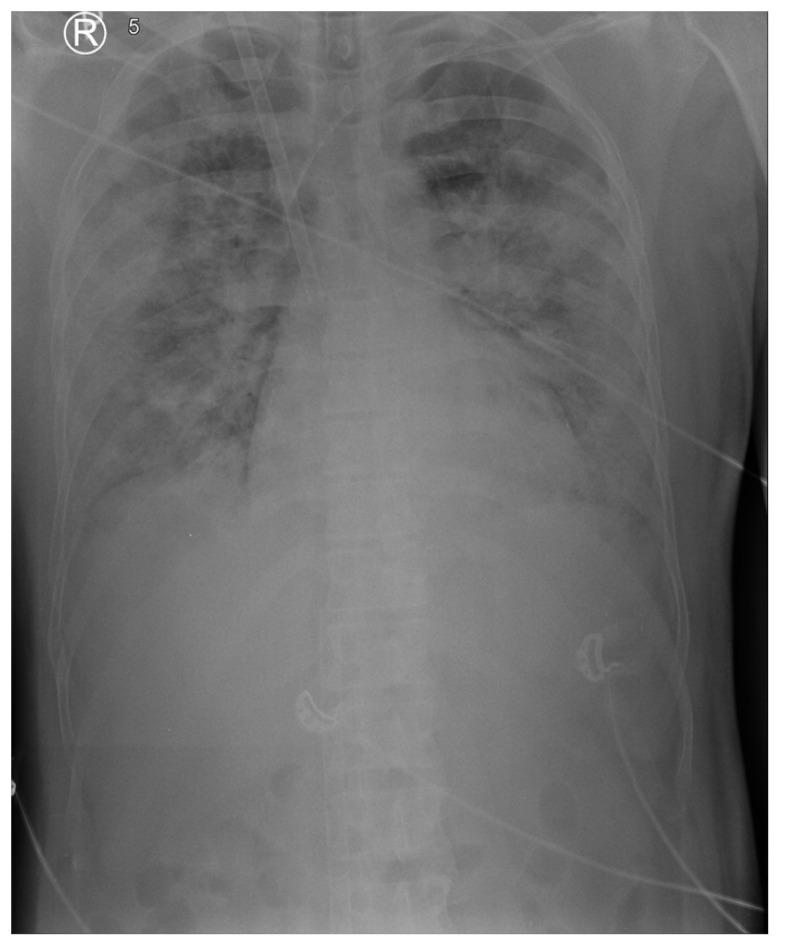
Chest X-ray on ECLS day 1. Chest X-ray revealed massive, confluent, bilateral opacities.
